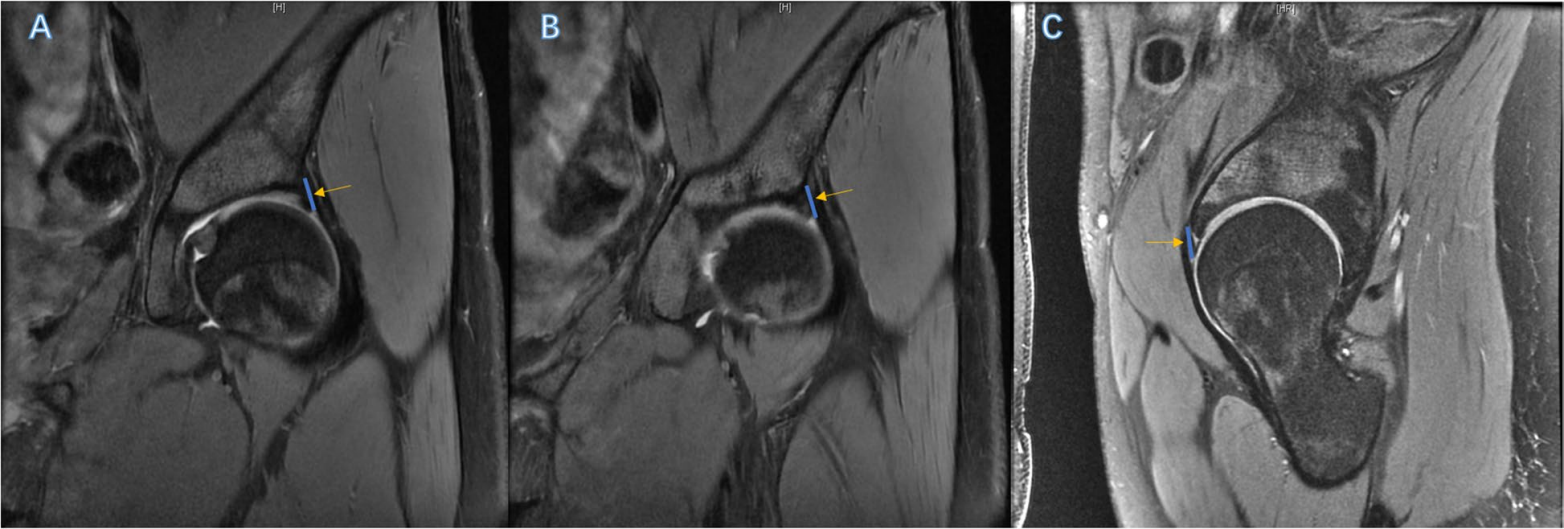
The measurement of the labral width in three standard positions in MRI scans. Legend: All three figures show the left hip. A The insertion of the indirect rectus (11:30) (B) The half-point of the indirect rectus insertion and the psoas U (1:30). C The psoas U (3:00).
